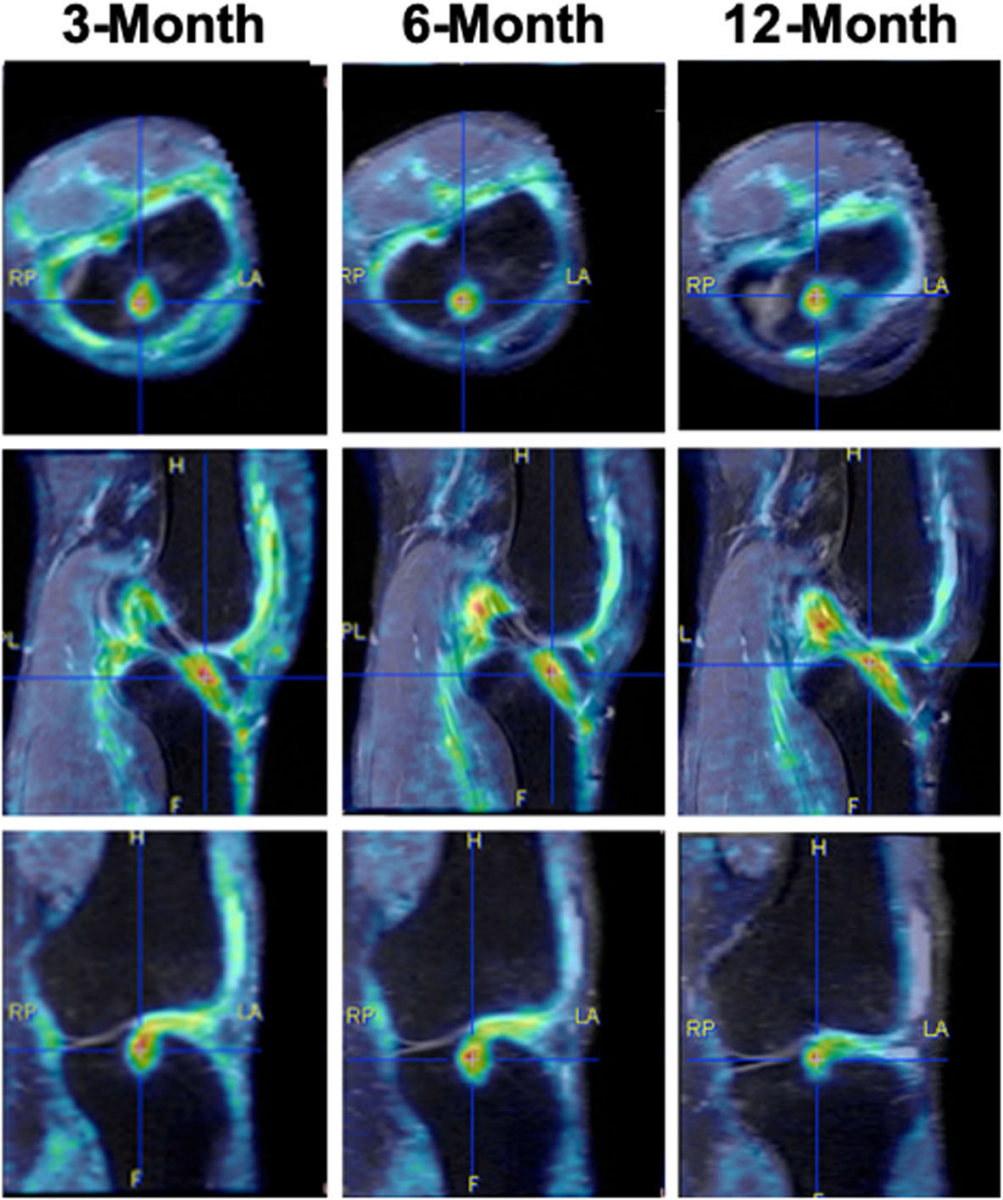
PET imaging shows focal areas of increased uptake. This figure shows three-month, six-month, and 12-month post-operative PET/MRI scans from the same patient scaled to same standardized uptake value (SUV) in the axial (top), sagittal (middle), and coronal (bottom) planes. Focal areas of high ^18^F-FDG uptake (red) are apparent, as well as an overall decrease in uptake over time
